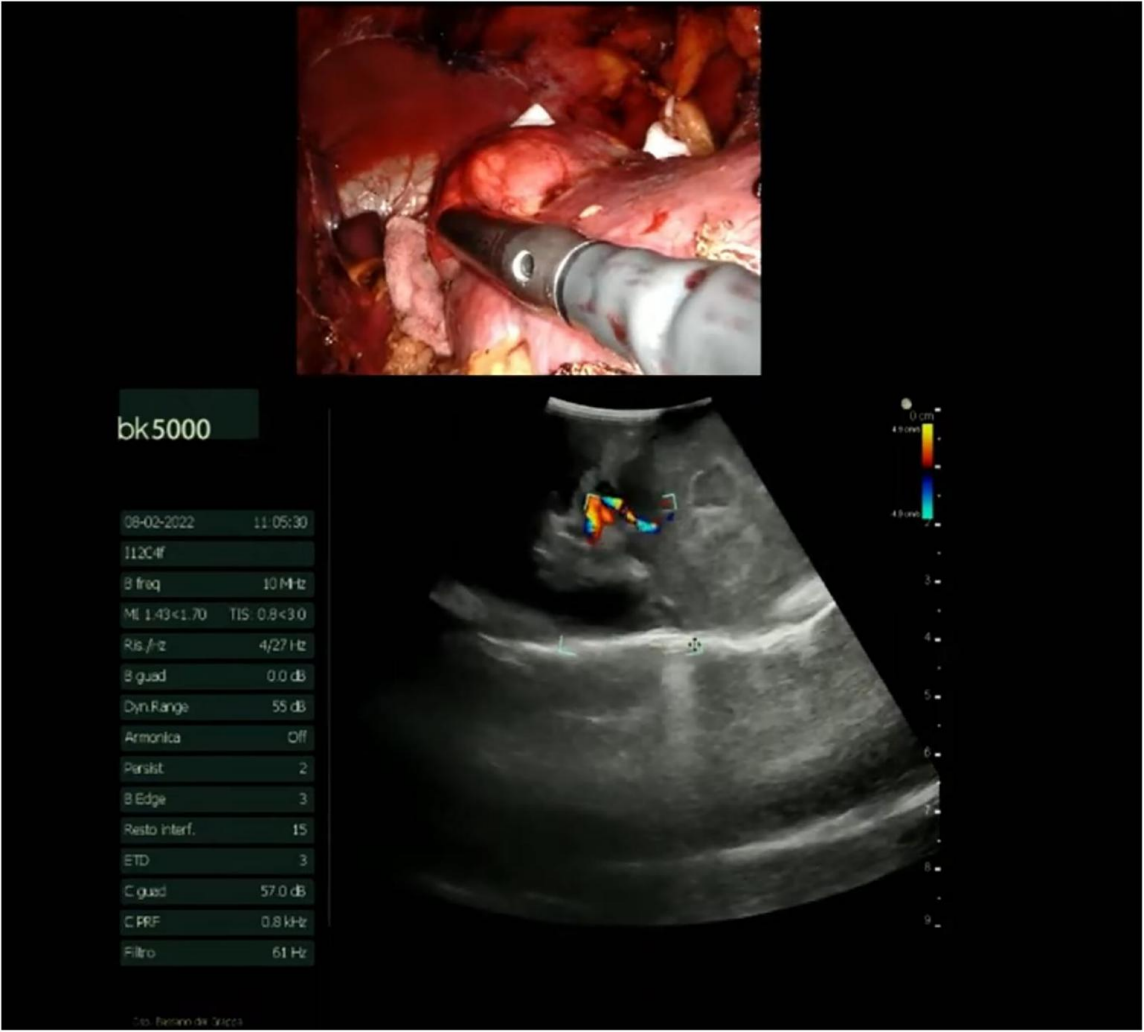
Intraoperative ultrasound.
